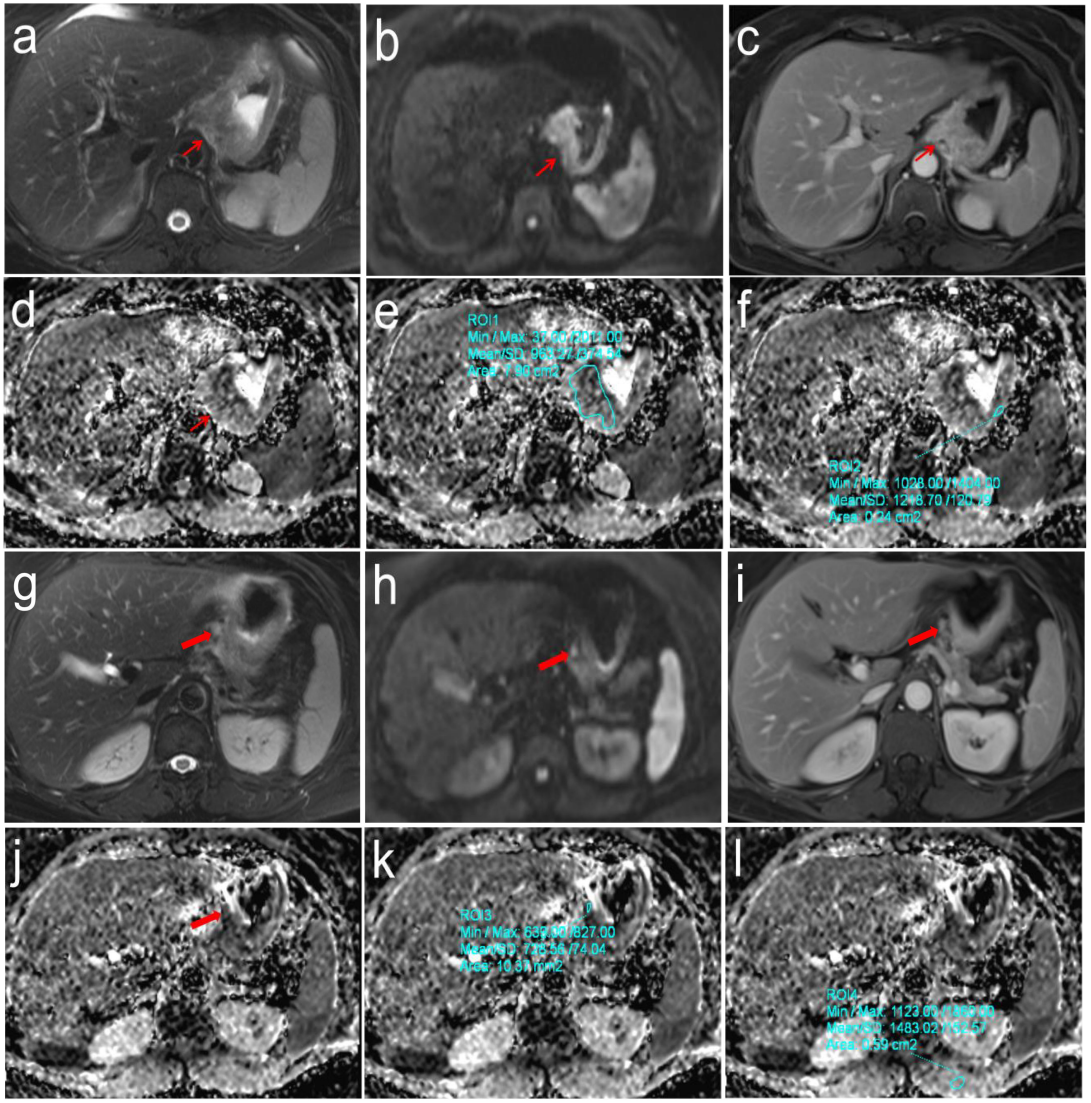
Pathologically confirmed gastric cardia adenocarcinoma (pT3N2M0) in a 57-year-old female. (a-f) T2WI, DWI and DCE images showed the location of primary tumor (Thin red arrow). The ADC values of primary tumor and normal gastric wall of the same axial section were 0.963 × 10^-3^mm2/s and 1.219 × 10^-3^mm2/s, respectively, the correspond rADCT value were 0.790; (g-l) T2WI, DWI and DCE images showed the location of perigastric sentinel lymph nodes (No. 2) (Thick red arrow). The short-axis diameter was about 6.2mm. The ADC values of target sentinel lymph nodes and the left vertical spinal muscle at the same axial section were 0.729 × 10^-3^ mm2/s and 1.483 × 10^-3^ mm2/s, respectively, the correspond rADCLN value were 0.492.
